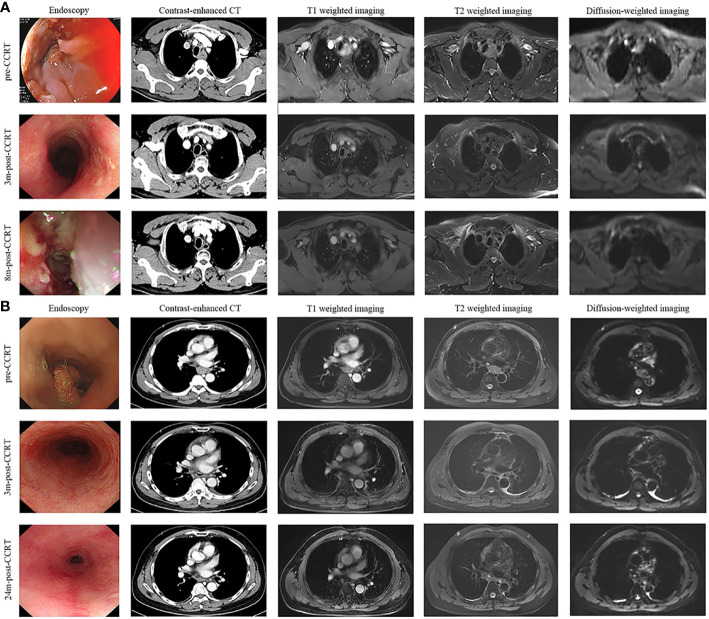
Disease evaluation by endoscopy, CT, T1-weighted, T2-weighted and diffusion-weighted sequences of MRI. (A) A 56-year-old, male patient was diagnosed with ESCC, which was located in the upper and middle thoracic esophagus. The treatment response of the case was assessed as CR after receiving CCRT. At 8 months after the completion of treatment, imaging examination showed no obvious abnormalities, but endoscopic examination revealed a small ulcerative lesion (red arrow) and pathological biopsy confirmed recurrence. (B) A 67-year-old, male patient was diagnosed with ESCC, which is located in the middle thoracic esophagus. The patient achieved CR after receiving CCRT and remained disease-free status in the 2-year follow-up period. *ESCC*, esophagus carcinoma; *CCRT*, concurrent chemoradiotherapy; *CR*, complete remission; *3m-post-CCRT*, 3 months after CCRT; *8m-post-CCRT*, 6 months after CCRT; *24m-post-CCRT*, 24 months after CCRT.
